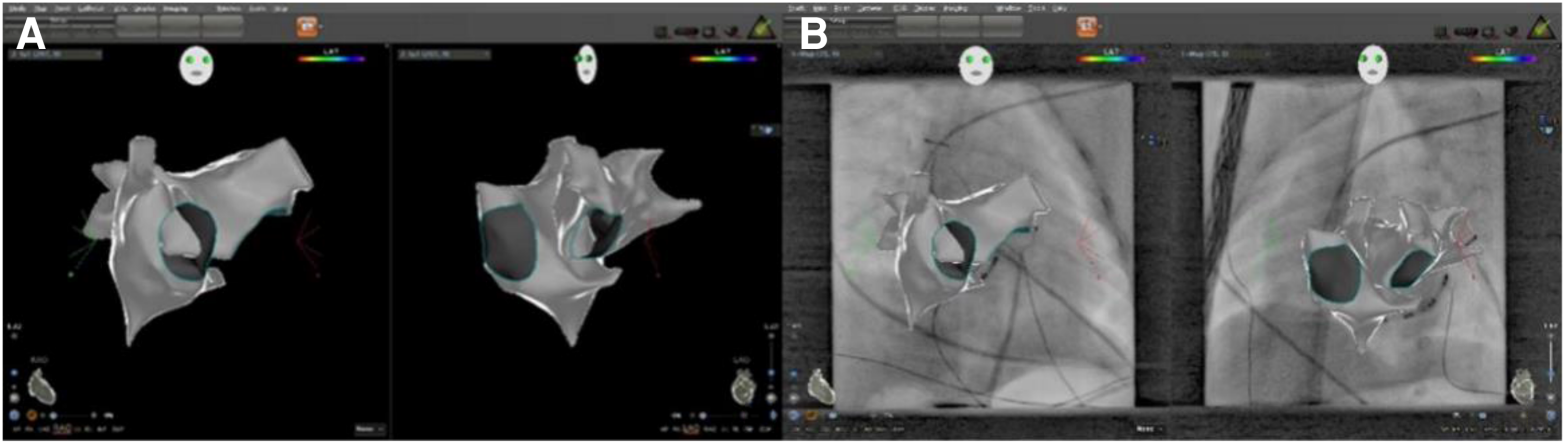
The cardiac 3D model images of CARTO3 and CARTO univu. Slide (A) shows the CARTO3 cardiac 3D model image with a right anterior oblique position of 30° and a left anterior oblique position of 45° on a black background; Slide (B) shows the CARTO Univu model, which puts the prerecorded x-ray cardiac image into the CARTO3 system and allows mapping and ablation on the background of x-ray cardiac images.
